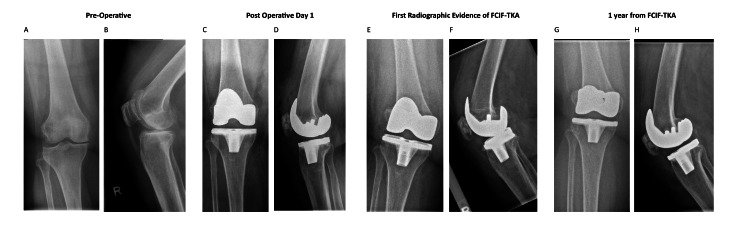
Patient 5 AP and lateral knee FCIF-TKA radiographs A: preoperative AP film, B: preoperative lateral film, C: postoperative day 1 AP film, D: postoperative day 1 lateral film, E: first radiographic evidence of FCIF-TKA AP film, F: first radiographic evidence of FCIF-TKA lateral film, G: one year from FCIF-TKA AP film, H: one year from FCIF-TKA lateral film AP: anterior-posterior, FCIF-TKA: femoral condyle insufficiency fractures following total knee arthroplasty
